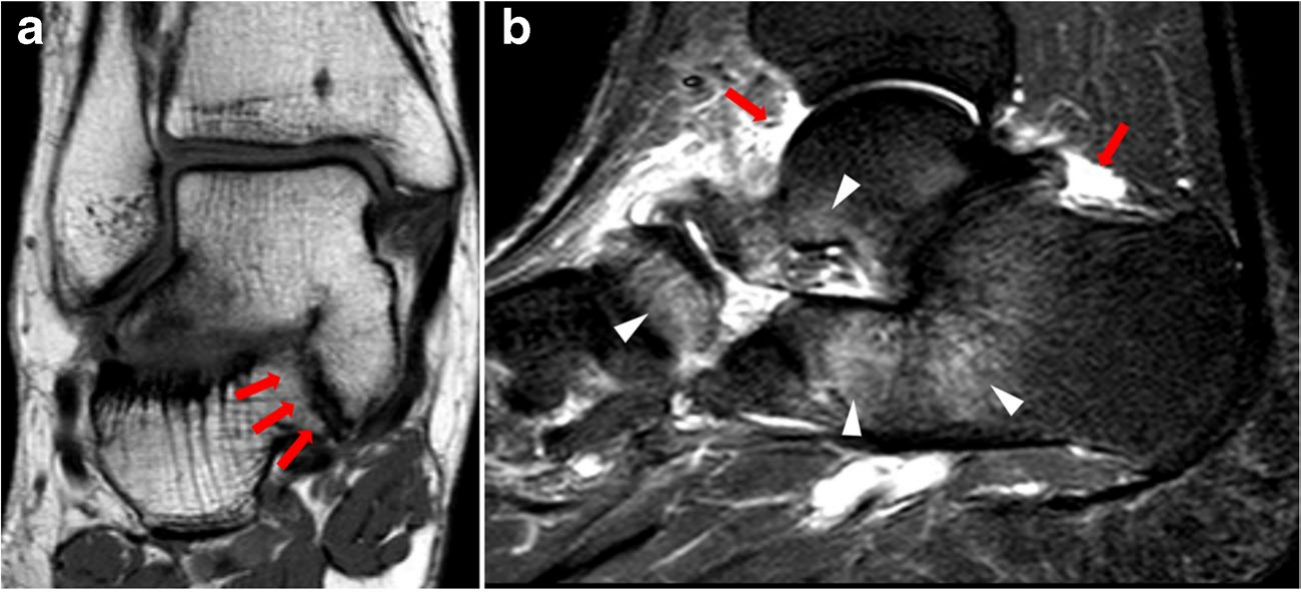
Coronal T1-weighted MR image of a 25-year-old male patient admitted for pain in the right foot diagnosed with talocalcaneal coalition in the middle facet (arrows, a), correctly diagnosed by all three readers. Sagittal STIR image in the same patient depicting diffuse bone marrow edema of the hindfoot (arrowheads) and joint effusion (arrows) (b)
